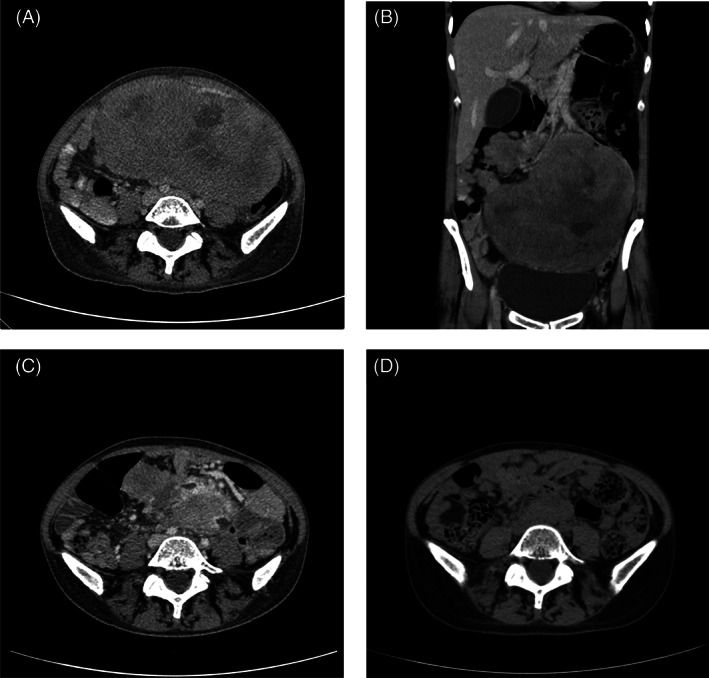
Case 1. (A,B) Contrast‐enhanced CT scan, at the onset of severe complication. (C) After 5 weekly cycles with MTX‐VA. (D) After a total of 42 cycles with MTX‐VA [Correction added on 12 July 2021, after first online publication: The size of Figures 1 and 2 has been updated in this version].
